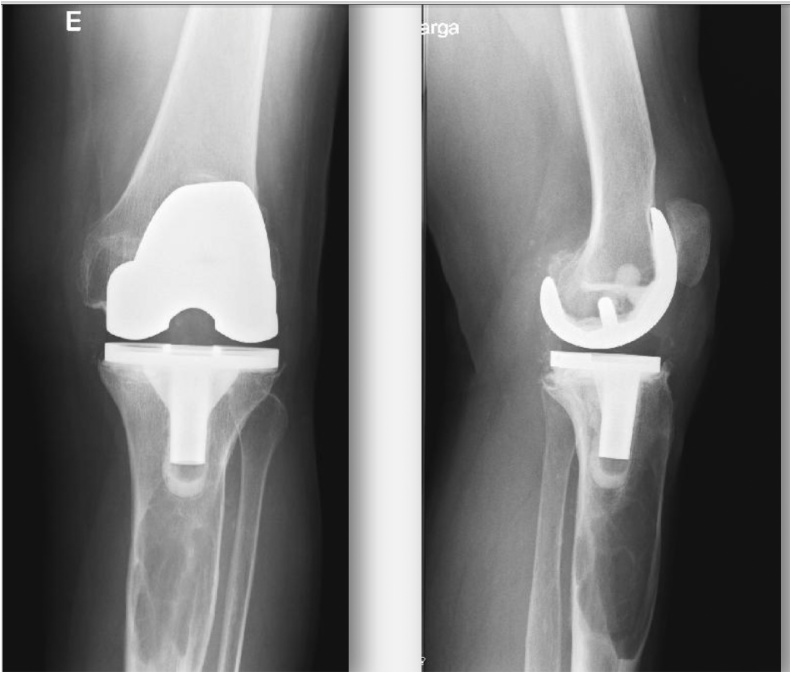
Antero-posterior and lateral x-ray view of the patient’s left knee. Please note the cystic lesion in the proximal tibial metaphysis.
